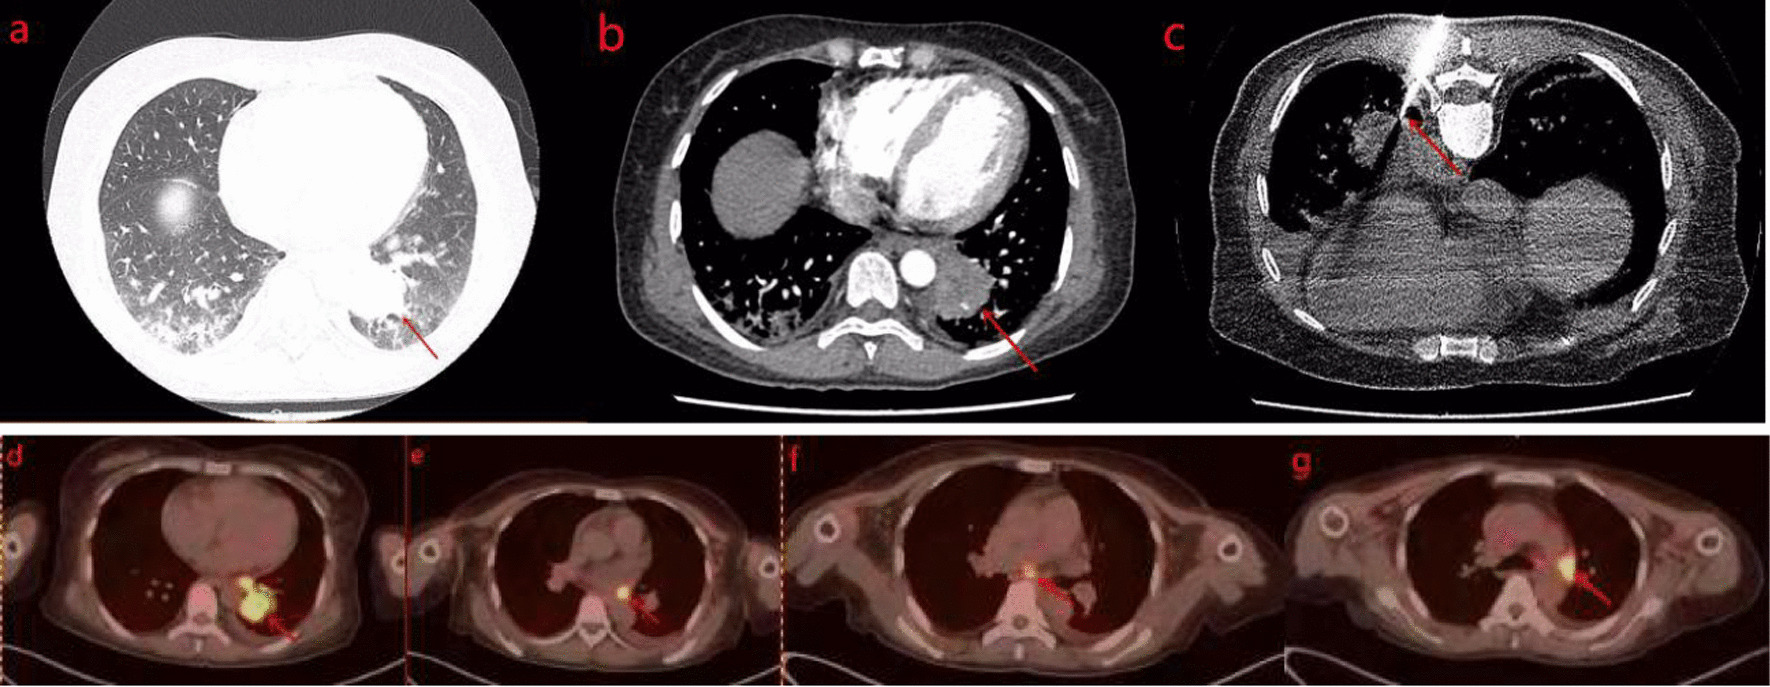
a Chest CT revealed a mass in the posterior basal segment of the left lower lobe (size 3.7 × 3.3 cm) with poorly-defined boundary, peripheral burr and lobulation sign. b Enhanced CT showed inhomogeneous enhancement of the mass with the branches of left inferior pulmonary artery and vein passing through. c CT-guided percutaneous transthoracic biopsy. d–g PET/CT showed SUV_max_ of the mass, the lpsilateral hilar and mediastinal lymph nodes were high
